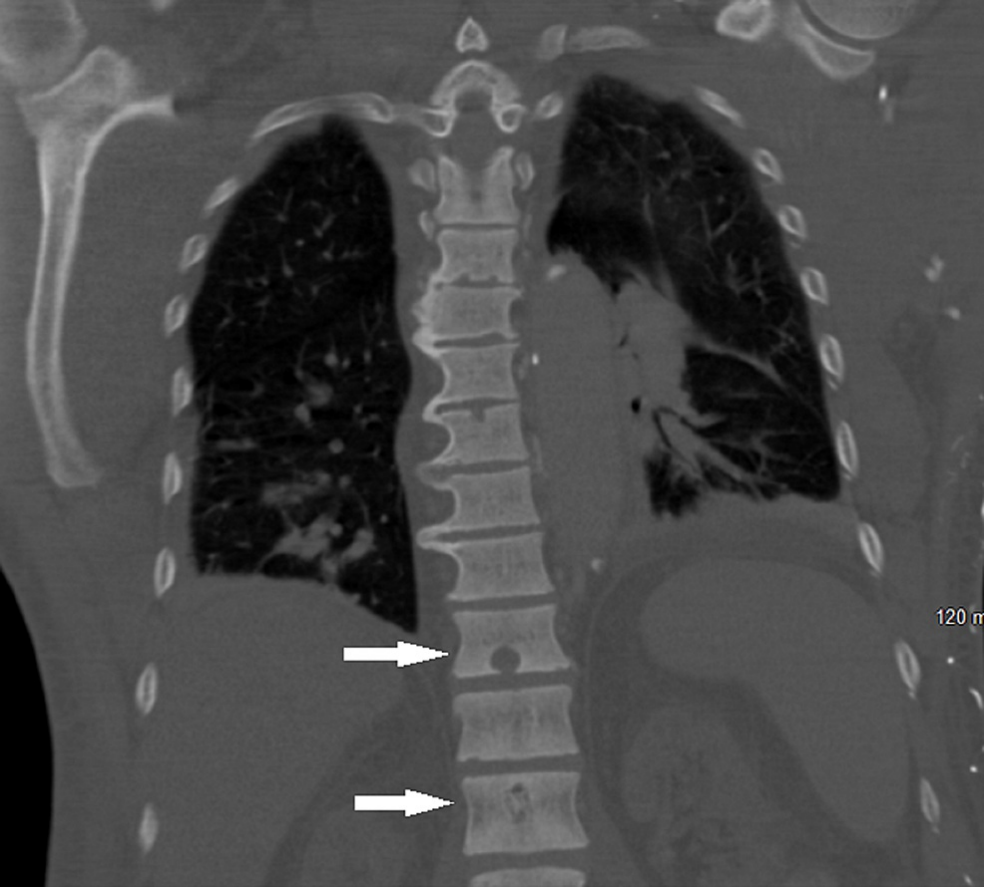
CT of the chest with contrast showing the bone lytic lesions in the thoracic vertebrae and L1 vertebra.
